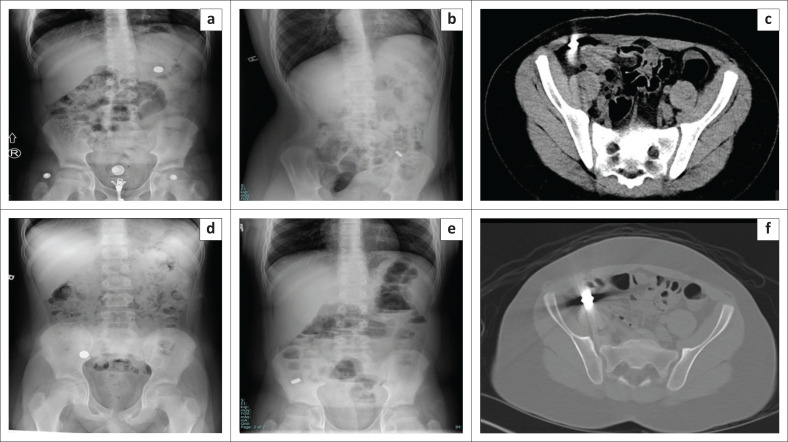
Serial abdominal radiographs and computed tomography (CT) images of a 12-year-old asymptomatic male child who swallowed five small magnets, shows the magnets initially in (a) the stomach, (b) then in the left lower quadrant and (c, d and e) later in the right lower quadrant. The multiplicity of magnets can be better appreciated on CT (c and f). The magnets were subsequently removed by colonoscopy.
